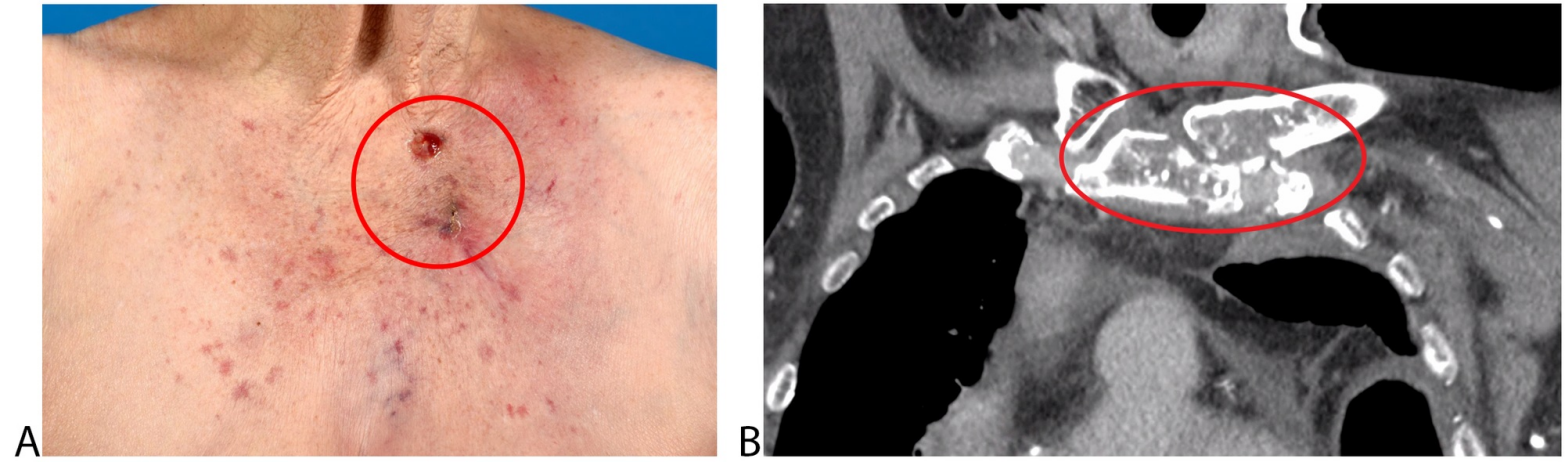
Ulcers based on osteonecrosis A) Physical exam of the two painful left parasternal ulcers; B) Coronal slice in a CT of the thorax made six years after initial radiotherapy showing destruction of the left sternoclavicular joint
